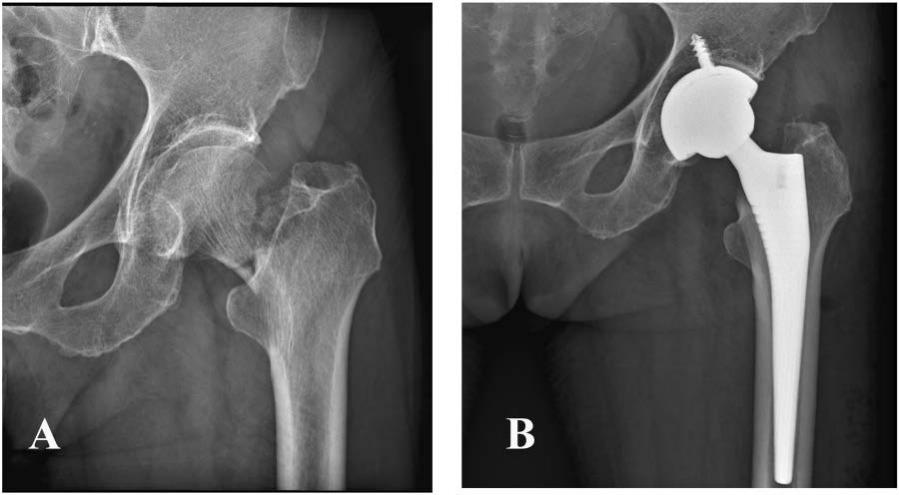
X.ray of a patient with a femoral neck fracture undergoing THA. Female, 80 years old, diagnosed with femoral neck fracture with sarcopenia (A) Preoperative X-ray (B) Postoperative X-ray.
